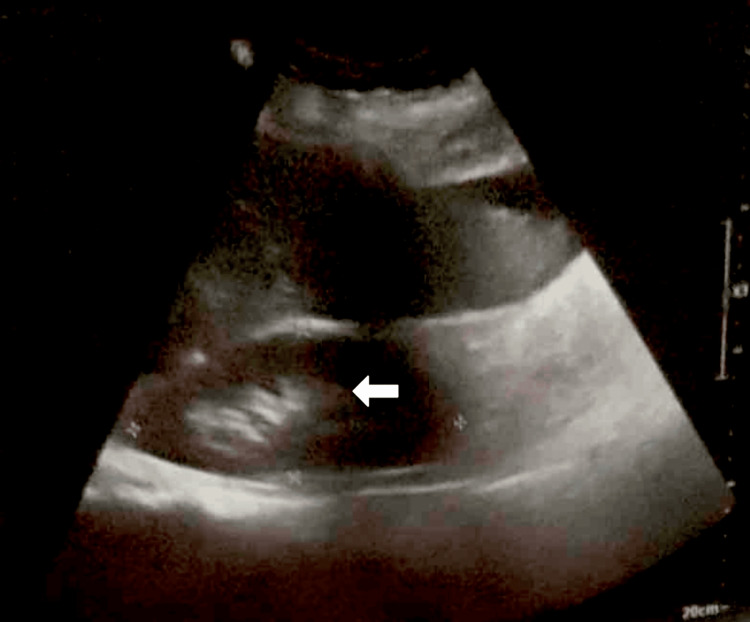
Ultrasound image of the right kidney demonstrating preserved corticomedullary differentiation and normal echotexture, indicating no structural abnormalities. The white arrow highlights the intact corticomedullary interface
